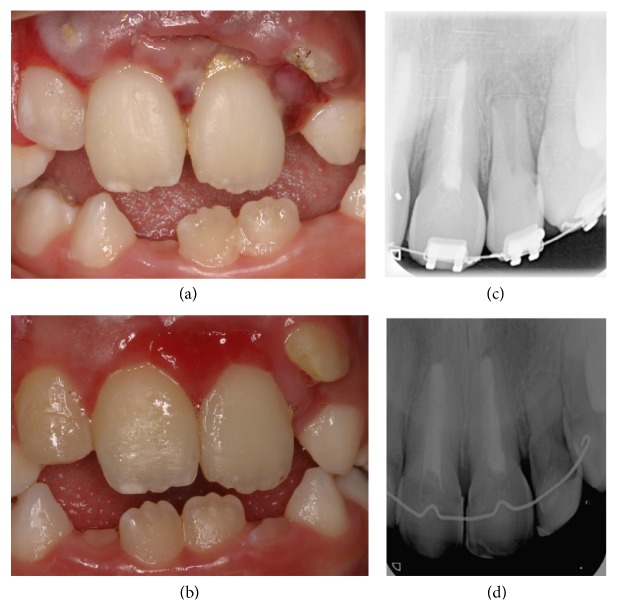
(a) Intraoral photograph of the patient at the clinic following trauma to the 4 incisors, with intrusion of the upper left lateral incisor. (b) Intraoral photograph of the patient following two weeks awaiting spontaneous reeruption of 22, showing insufficient reeruption. (c) Periapical radiographic projection of the orthodontic extrusion carried out after the failure of spontaneous reeruption, with complete extrusion of 22 and root development visibly indicated. (d) Radiographic projection 6 years after the trauma, in which arrested root growth and bone tissue growing inside the root canal can be observed on one of the control periapical radiographs taken after orthodontic treatment.
